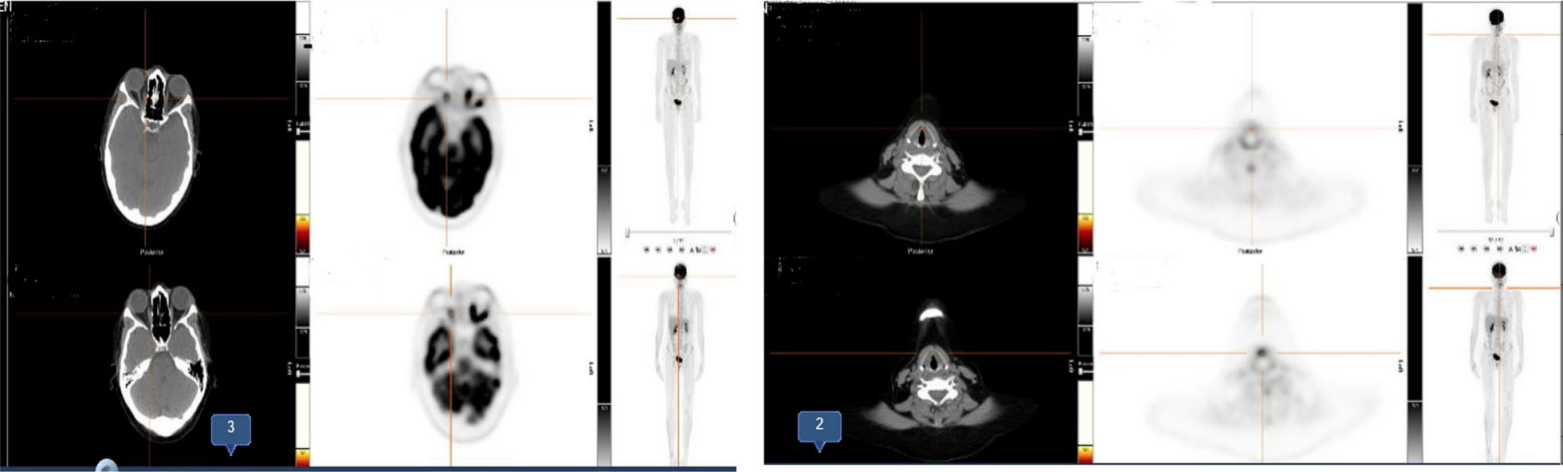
Fluorodeoxyglucose- positron emission tomography (FDG-PET) images of patient, before starting pembrolizumab. FDG PET shows a discrete focus of hypermetabolism in the medial rectus muscle on the right corresponding to the area of greatest thickening, suggestive of residual lymphoma as physiologic activity in the other extraocular muscles on the right is absent. There is a focal area of hypermetabolism within the anterior soft tissues adjacent to the intrinsic laryngeal musculature in keeping with viable lymphoma in laryngeal area
